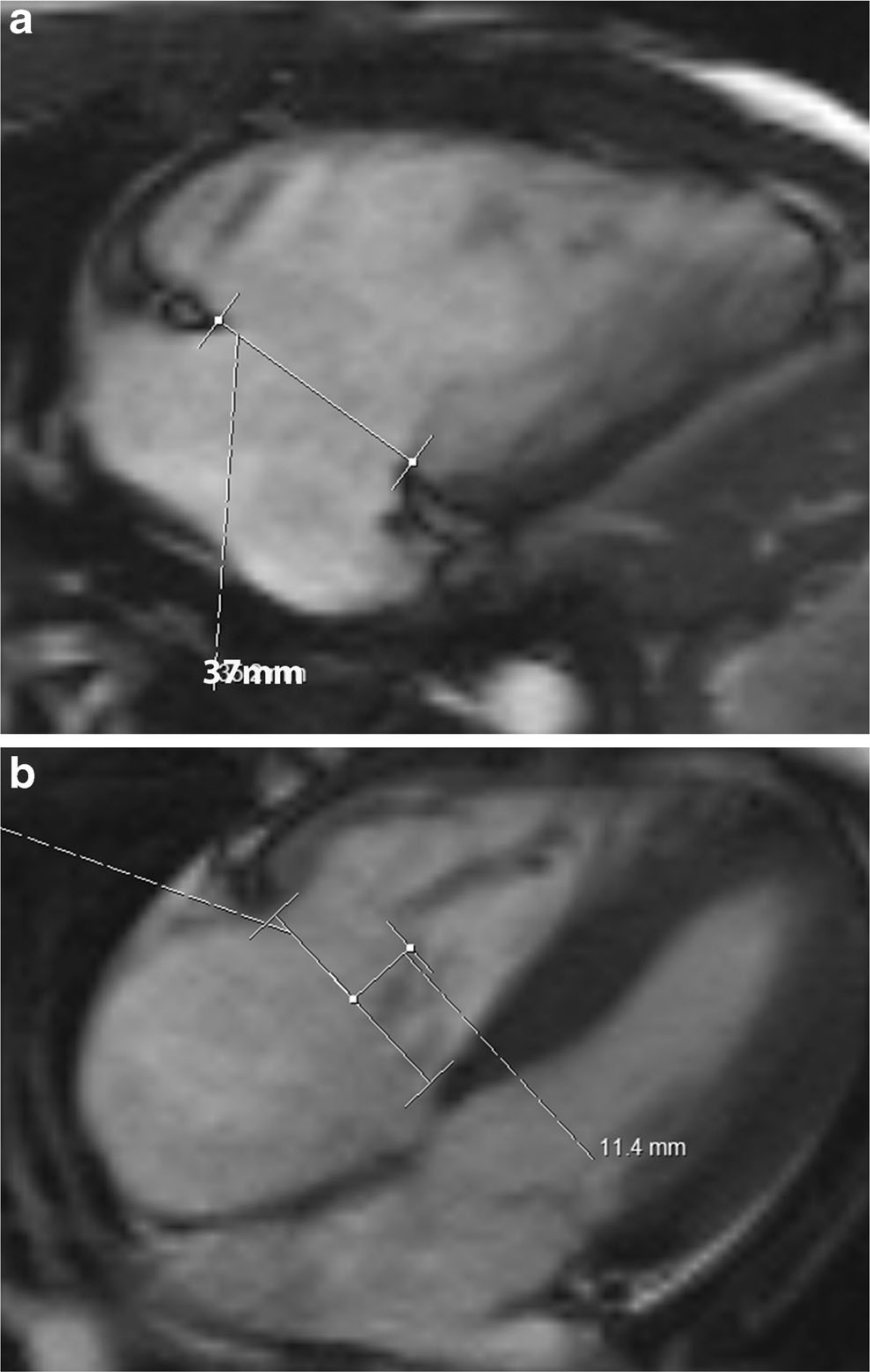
Tricuspid regurgitation (TR). (a) Four-chamber steady-state free precession (SSFP) image in a patient with functional TR shows dilation of the annulus > 35 mm. The annulus is also flat and planar. Dilation occurs along the free wall of the tricuspid annulus. The septal wall is intact. (b) Tethering. Four-chamber SSFP MRI image shows distance from the annular plane to coaptation in systole > 8 mm and area > 1.6 cm^2^, predicting residual TR following surgery
